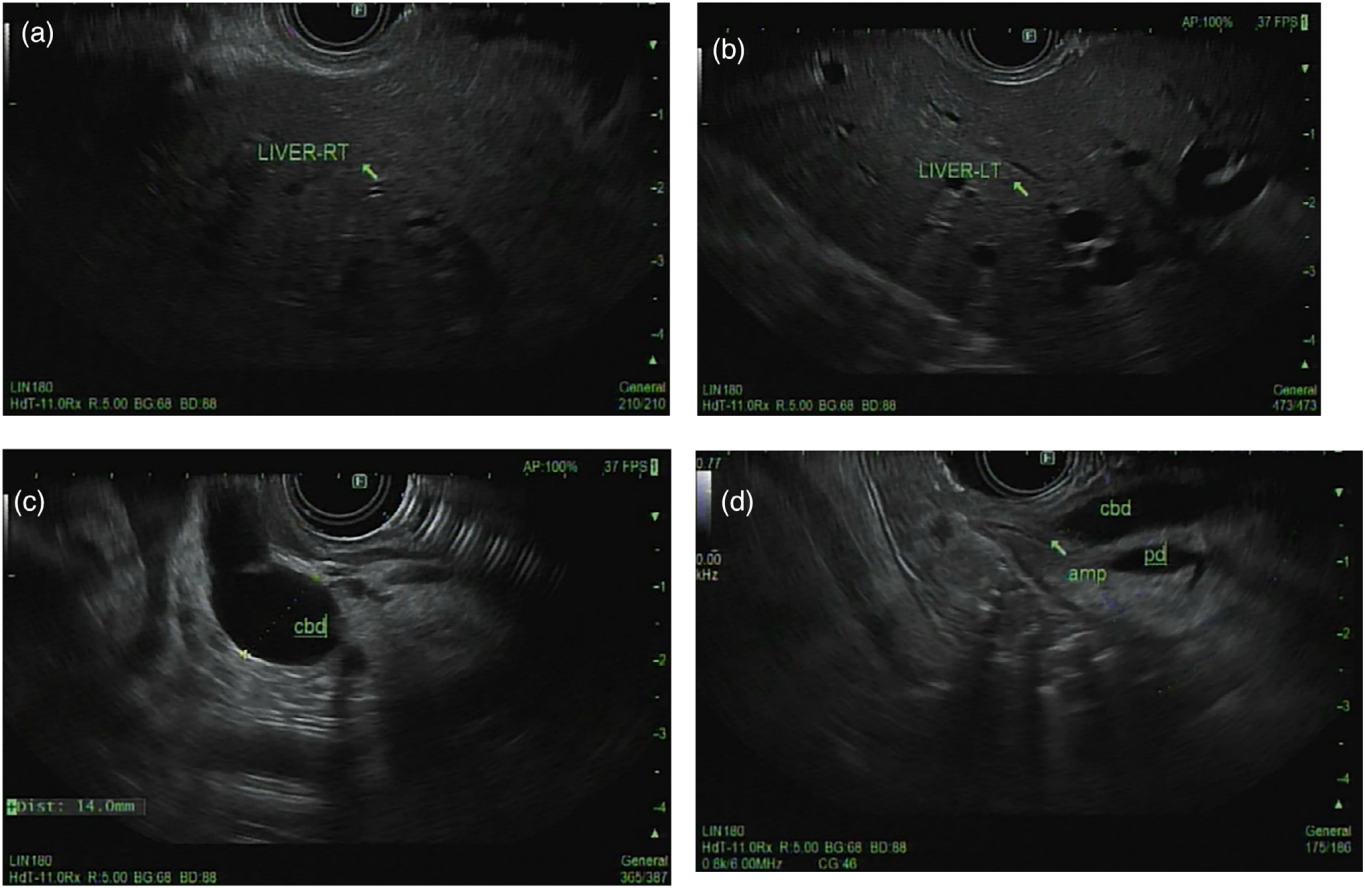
Endoscopic ultrasound (EUS) prior to biopsy showing normal Right (a) and Left (b) liver lobe parenchyma with dilated biliary tree and common bile duct (CBD) > 13 mm and visible ampulla (c, d).
